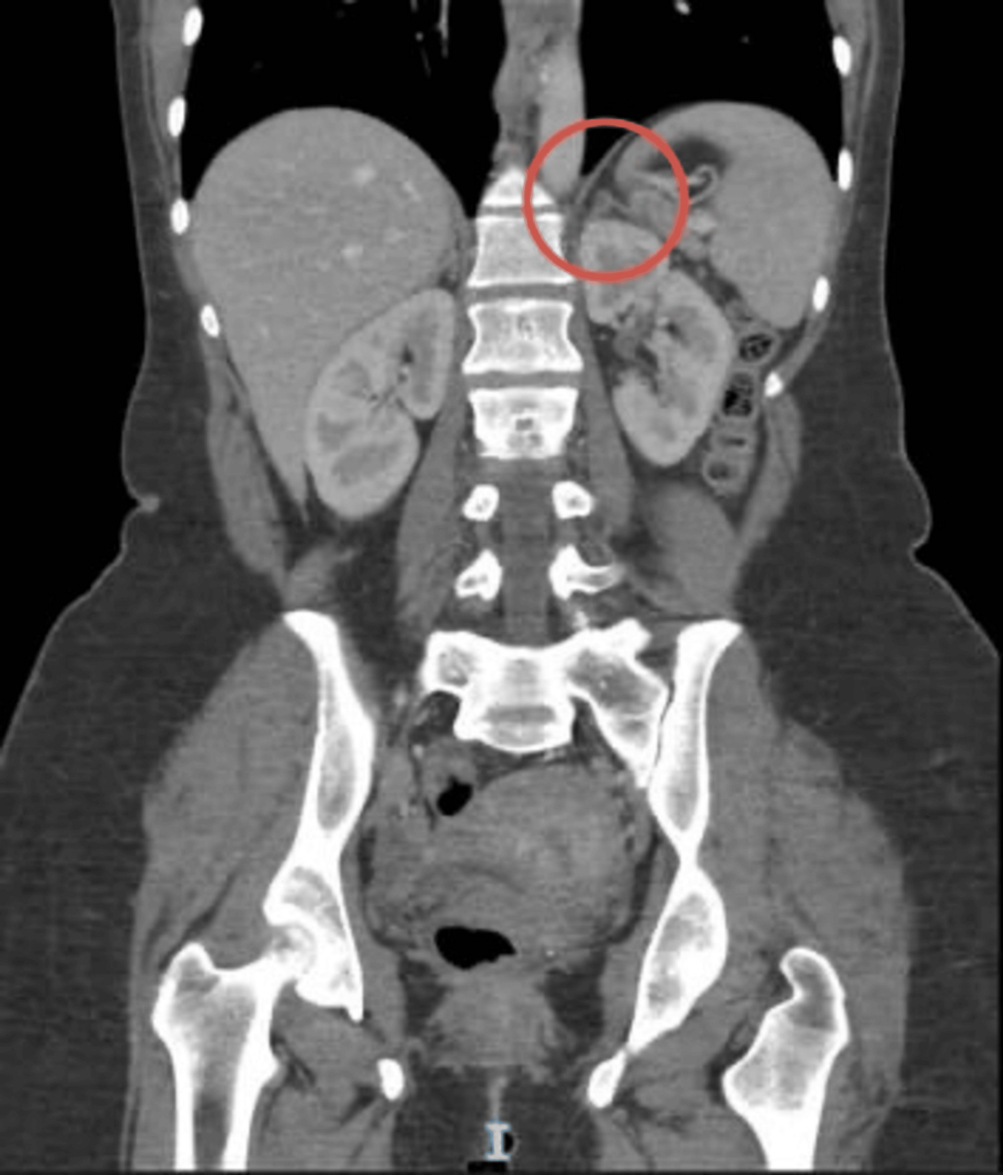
CT abdomen showing nodular thickening of the left adrenal gland (circled) CT, computed tomography
